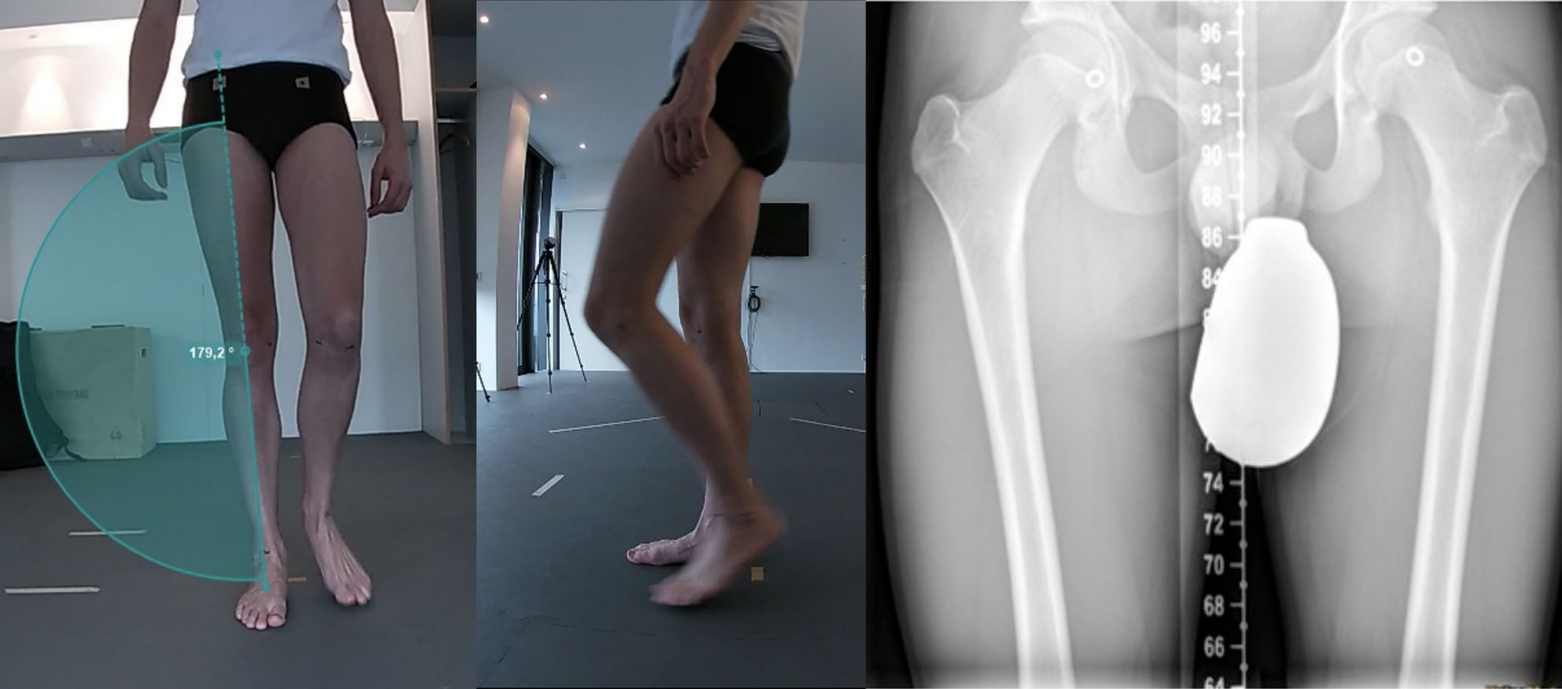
Mechanical leg axis/HKA determination based on anatomical landmarks, midstance gait analysis in two planes, and radiographic verification of the hip joint center
